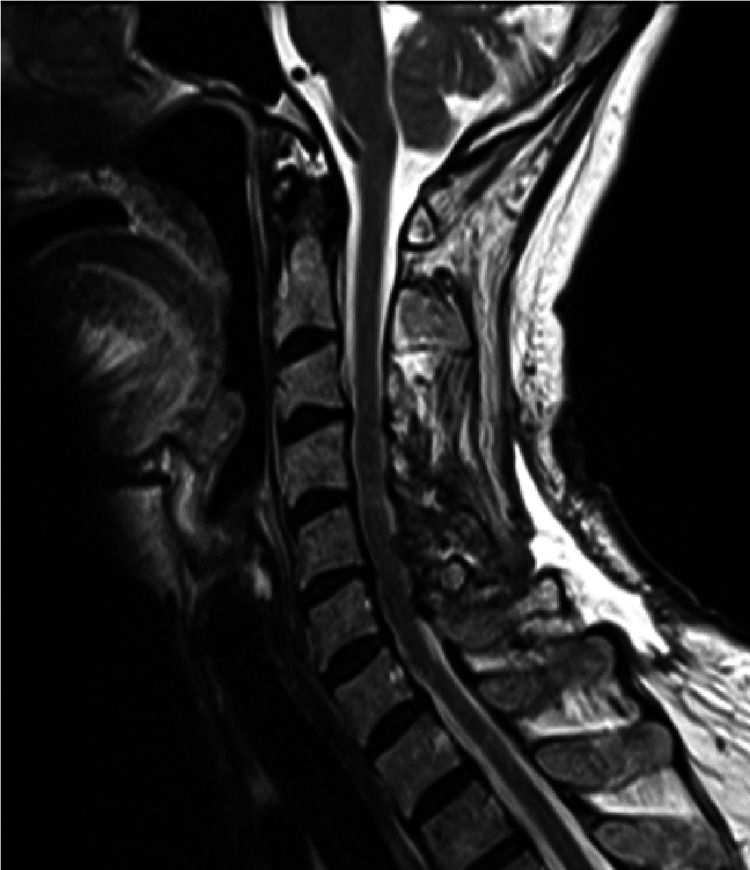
Case 1 postoperative cervical sagittal T2-weighted MRI image
